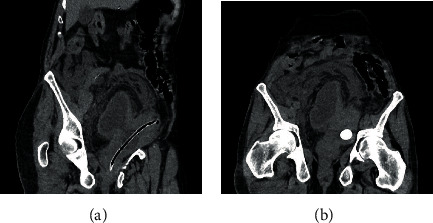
(a, b) Coronal oblique reconstruction of the CT scan showing collapsed bladder with a large diverticulum arising from the right superolateral bladder wall, with a large bladder stone and Foley catheter in place, associated to a small amount of free fluid in the peritoneal cavity and around the diverticulum, with an enlarged prostate gland.
